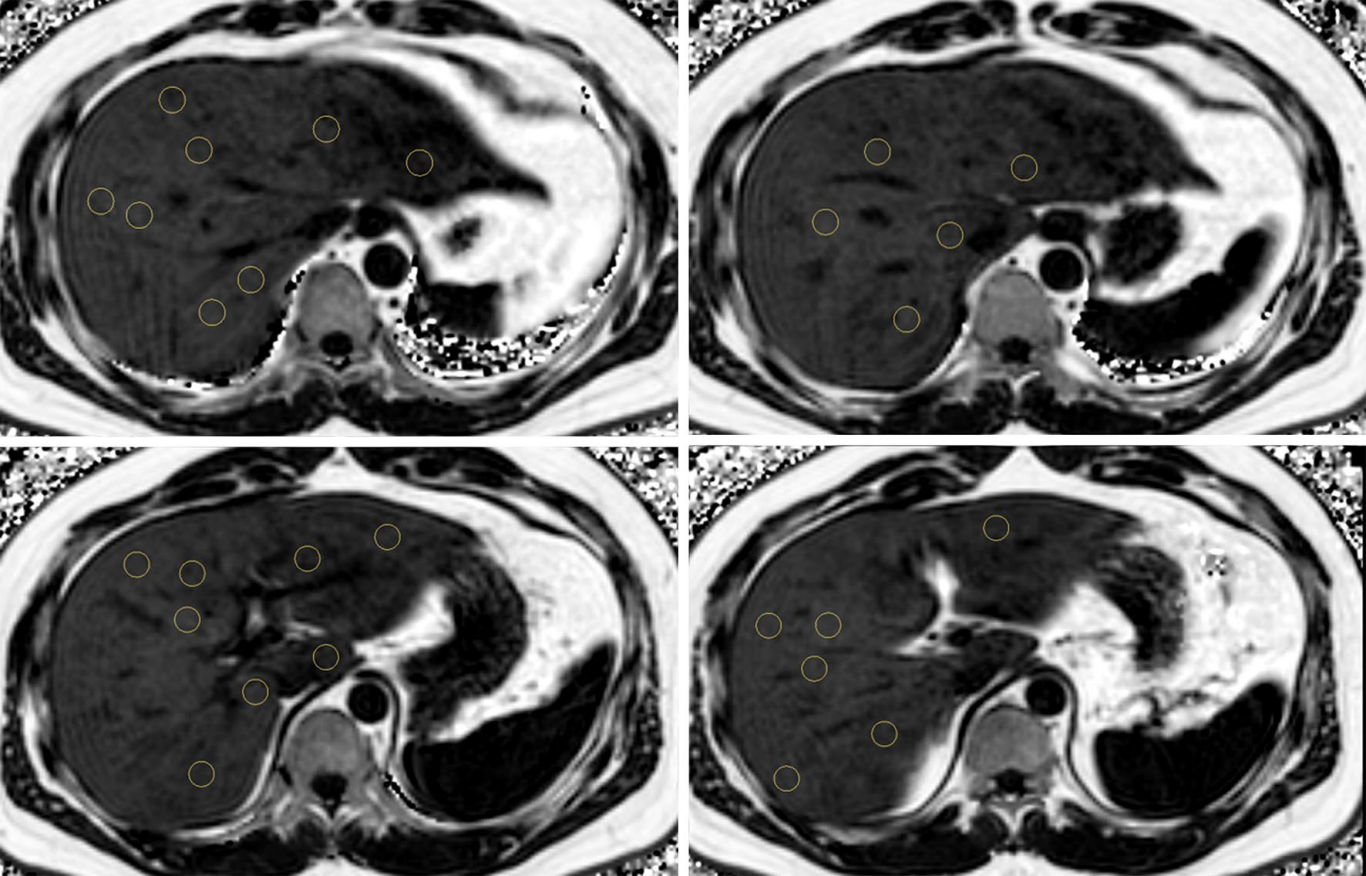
A demonstrative image of magnetic resonance imaging-proton density fat fraction (MRI-PDFF) measurement. Three circular regions of interest (100 mm^2^ area) were drawn in each hepatic segment to avoid large vessels, bile ducts, capsules, and space-occupying lesions. The average of 24 measurements in each hepatic segment was considered the mean MRI-PDFF value.
